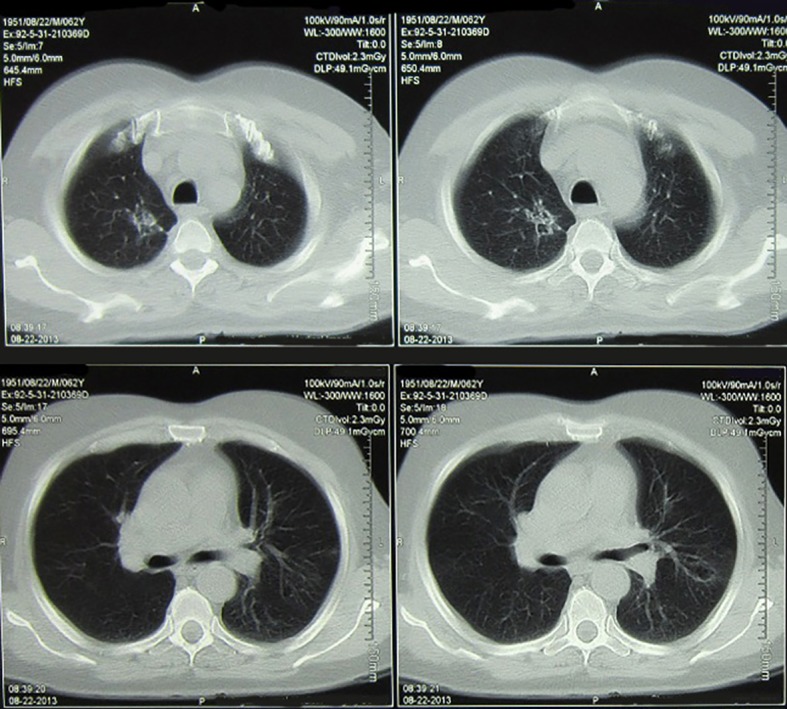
Shows the chest CT scan of the patient after the 3rd cycle of chemotherapy with cabazitaxel.
